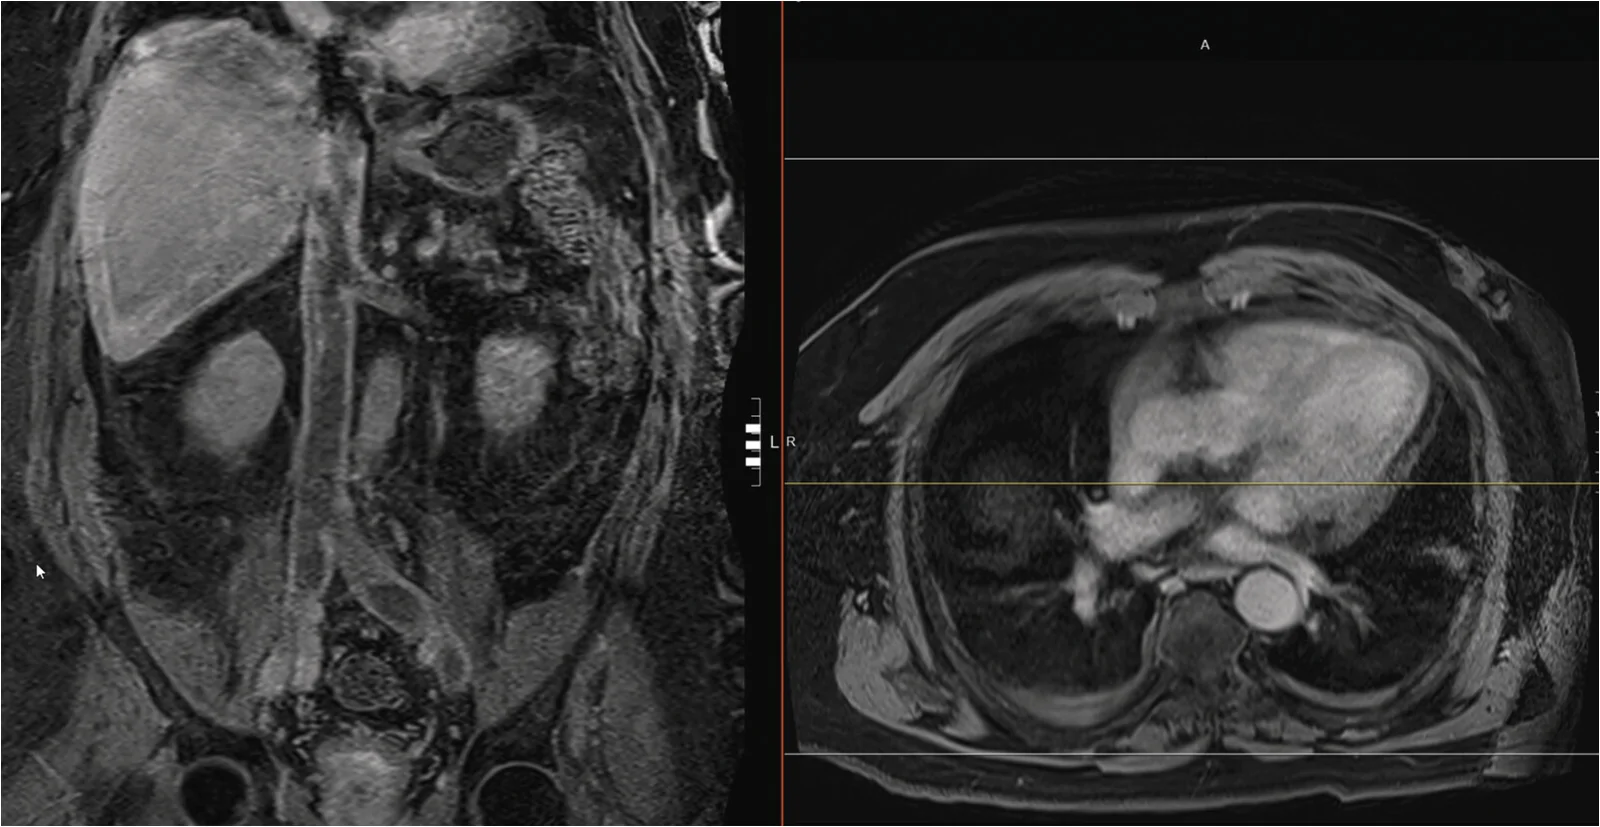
Abdominal MRI showing extent of thrombus.
